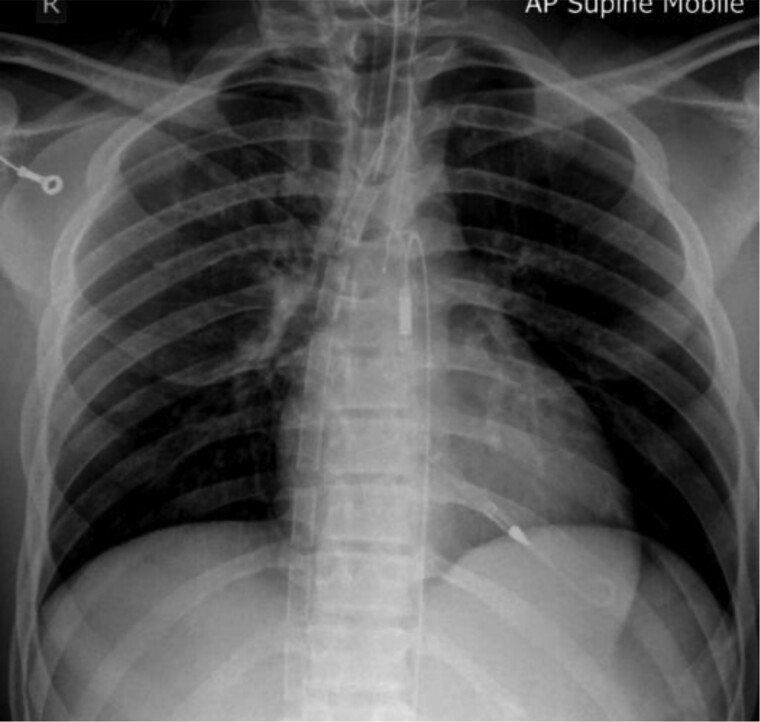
Chest radiograph showing extracorporeal membrane oxygenation cannula position and Impella device.
